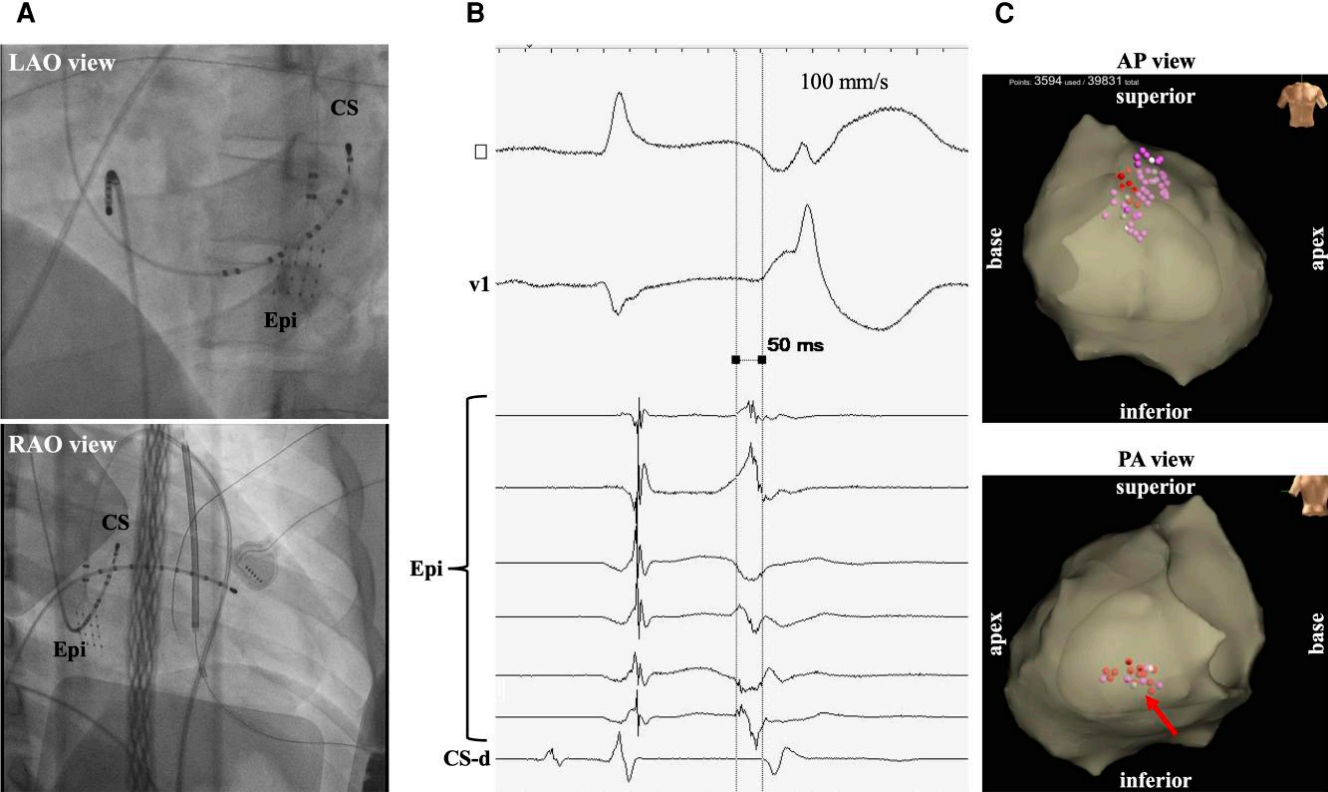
(*A*) X-ray imaging during premature ventricular contraction cauterization. (*B*) The local prepotential at the ablation point was 50 ms ahead of the premature ventricular contraction. (*C*) Ablation site indicated by three-dimensional mapping. The arrow shown on the left ventricle posterior wall corresponds to the earliest site of premature ventricular contraction.
